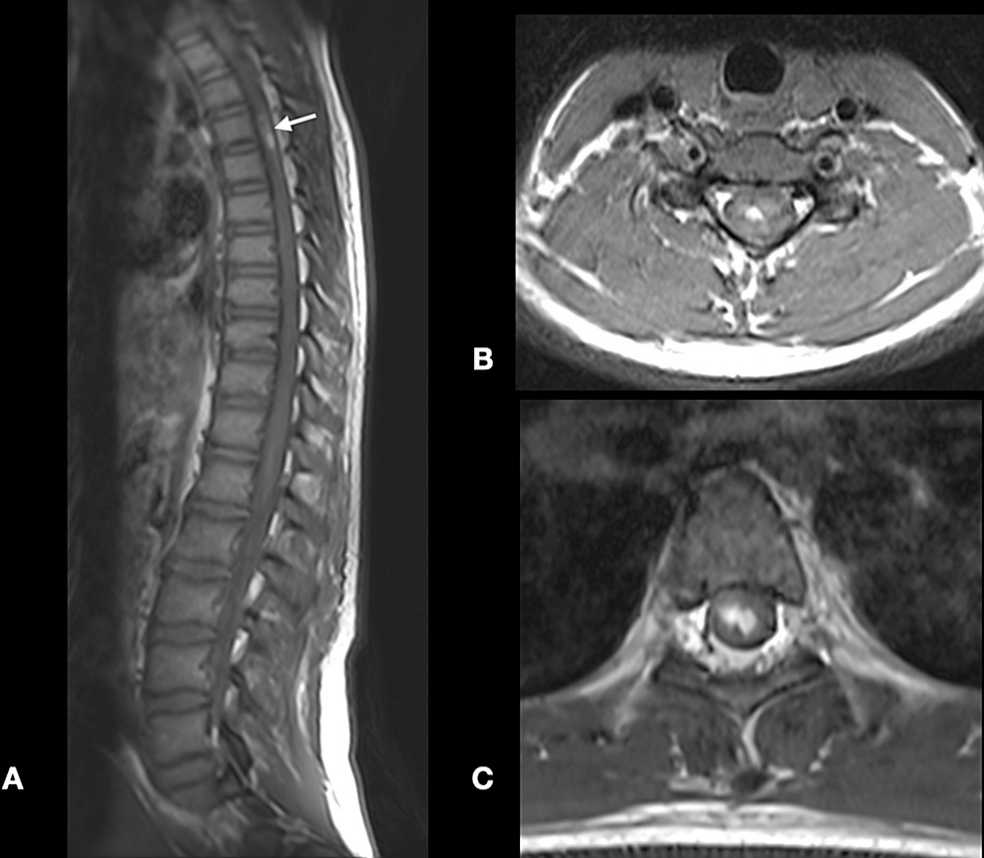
Sagittal T1-weighted MRI scans of the thoracic spine (A) and axial T2-weighted MRI scans (B, C) revealed the signal changes suggestive of subacute hemorrhagic changes (white arrow).
